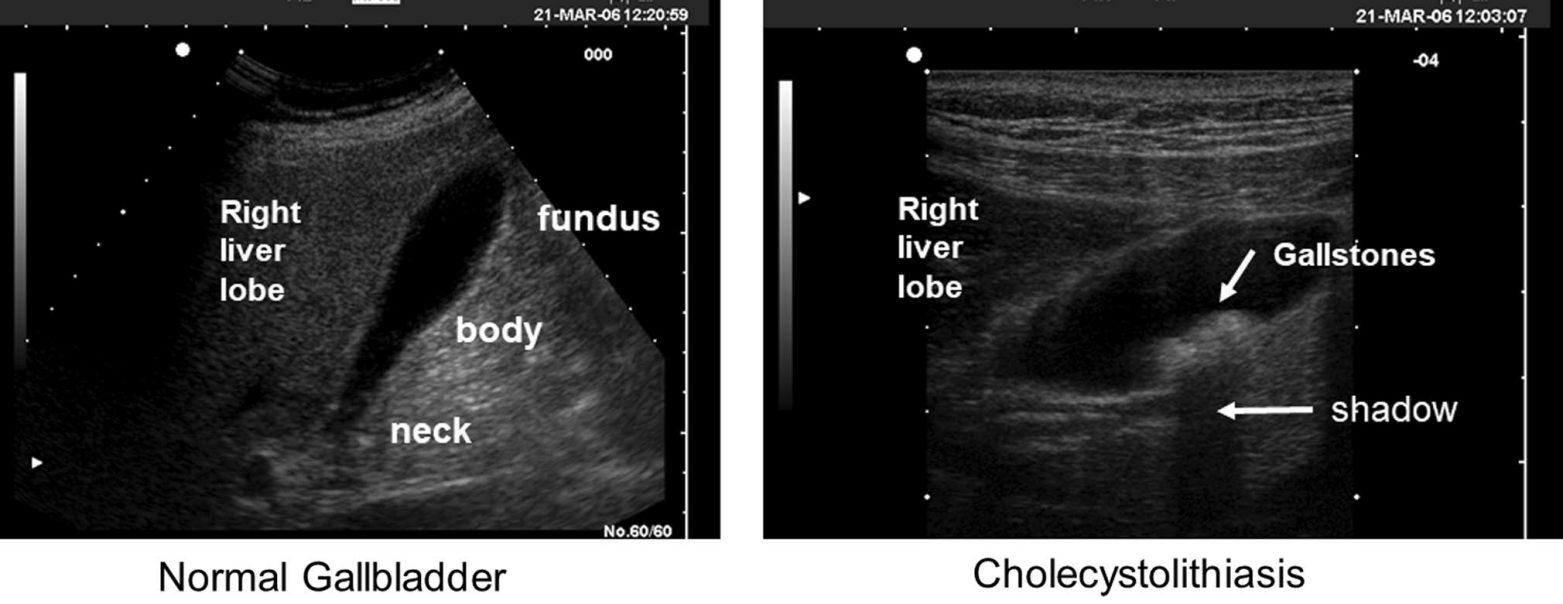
Ultrasonographic study of the gallbladder in the fasting subjects using a 3.5 MHz probe and the longitudinal scan. In the normal gallbladder the content in the lumen (bile) is totally anechoic (i.e., gallstone free and sludge free). The gallbladder wall is slightly hyperechogenic and less than 3 mm thick. The gallbladder has a length of ~ 90 mm and depth of ~ 22 mm. By assuming that width is same size of depth, the fasting gallbladder volume is estimated to be 22.8 mL (ellipsoid formula). In cholecystolithiasis the longitudinal scan shows at least 2 hyperechoic rounded spots each ~ 5–6 mm in size and layering on the distal gallbladder wall. The spots are mobile and gravity dependent at real-time examination and represent stones. The anechoic shadow departs from the distal border of the stones due to posterior attenuation of the ultrasonographic beam
